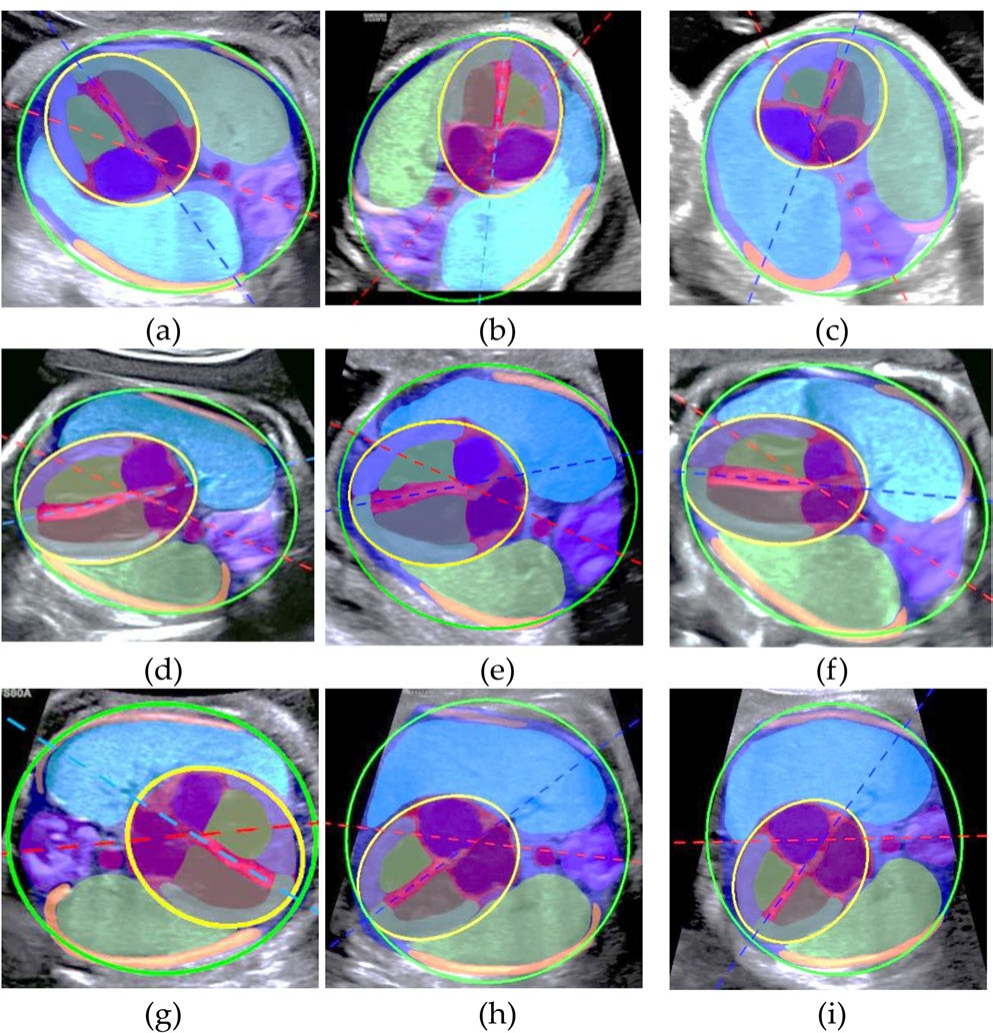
AI-based model measurements of cardiac parameters from various positions. (a)–(c) Apical fetal four-chamber view; (d)–(f) Parasternal fetal four-chamber view; (g)–(i) Basal fetal four-chamber view
